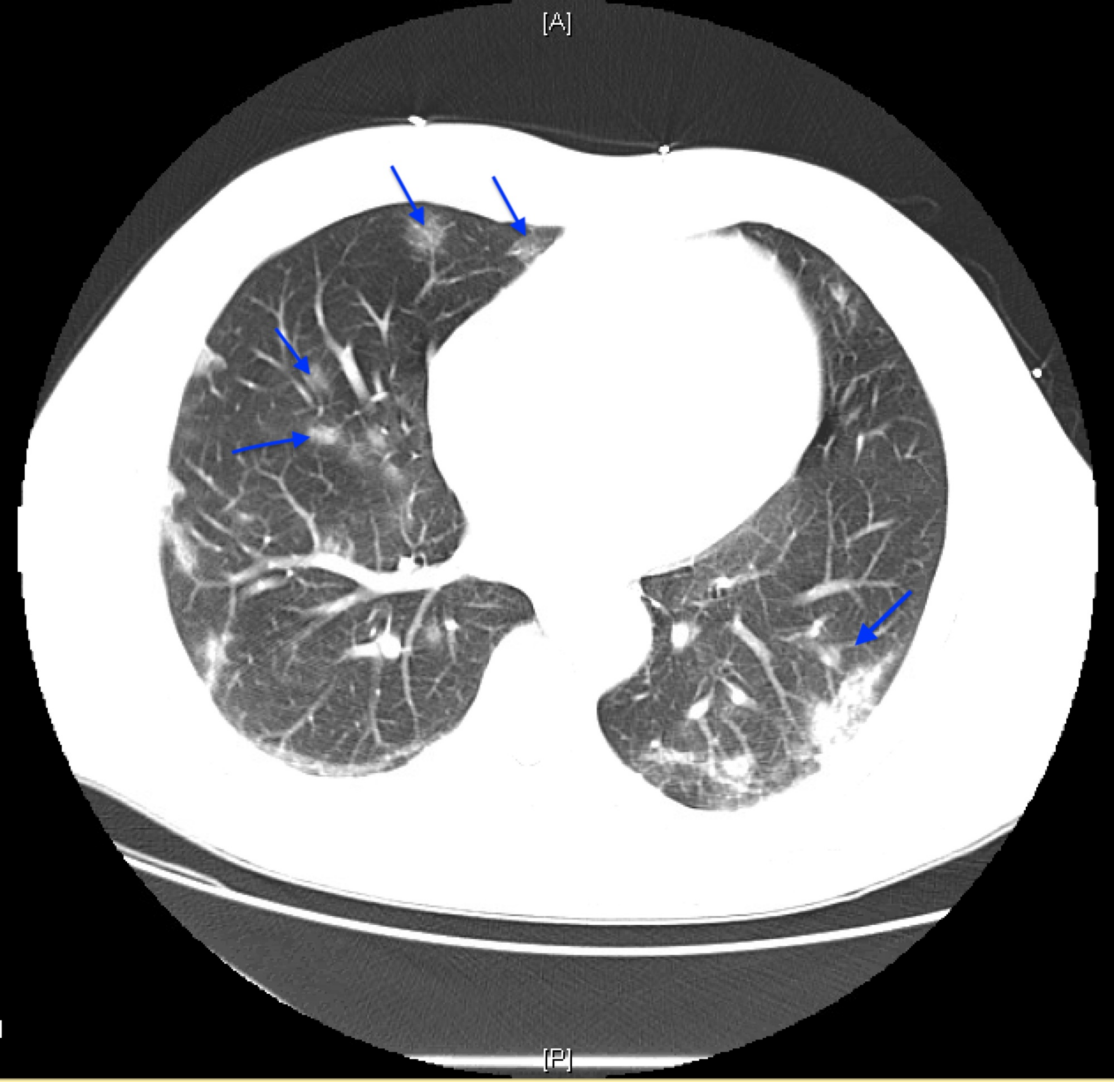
Non-contrast chest CT demonstrating patchy subpleural multifocal ground-glass opacities involving the bilateral upper and lower lobes (blue arrows).
